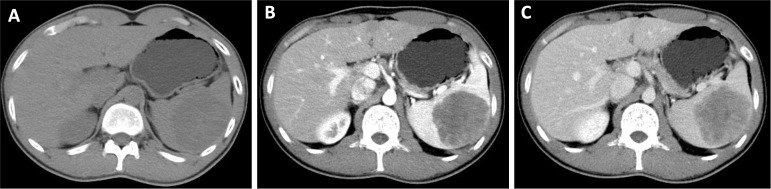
A 21-year-old man with a splenic mass diagnosed as papillary intralymphatic angioendothelioma. A plain abdominal CT scan (A) reveals an isodense mass in the spleen, showing mild enhancement in the arterial phase (B) and further enhancement in the venous phase (C).
